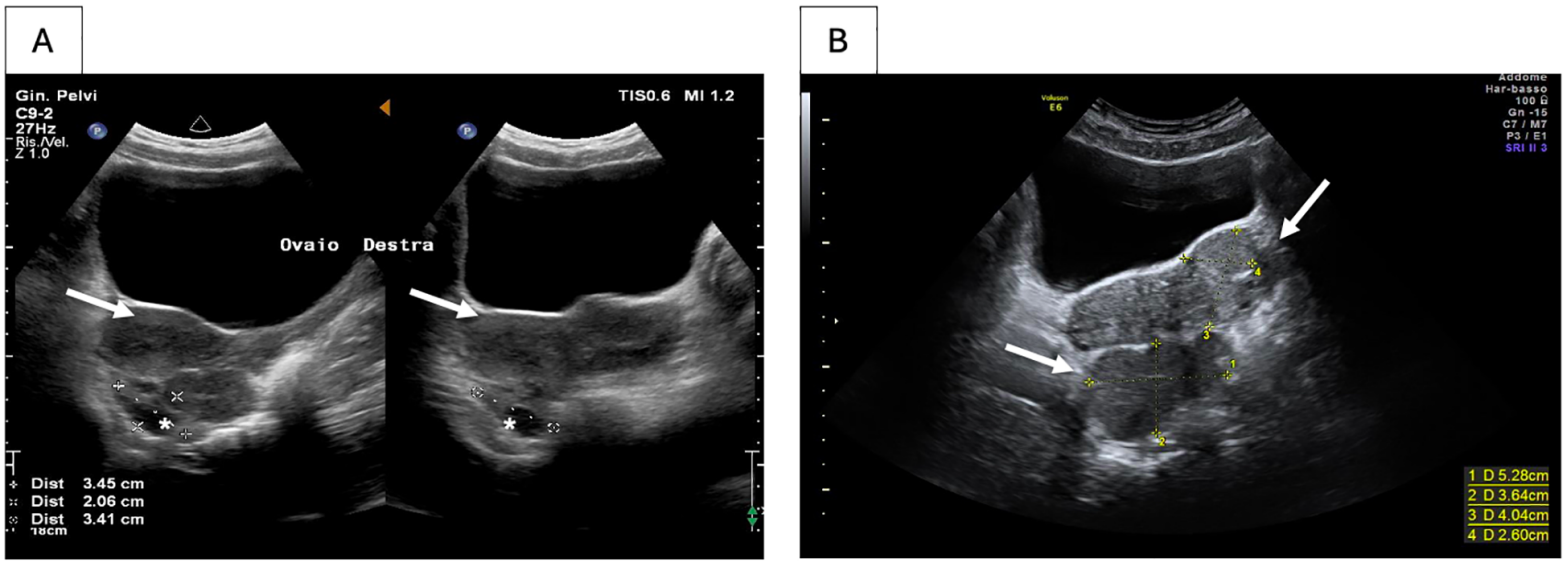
Ultrasound of the pelvic splenosis. Splenosis (A) at diagnosis and (B) six months after diagnosis. White arrows indicate the ectopic splenic tissue. Right ovary is shown by the *.
